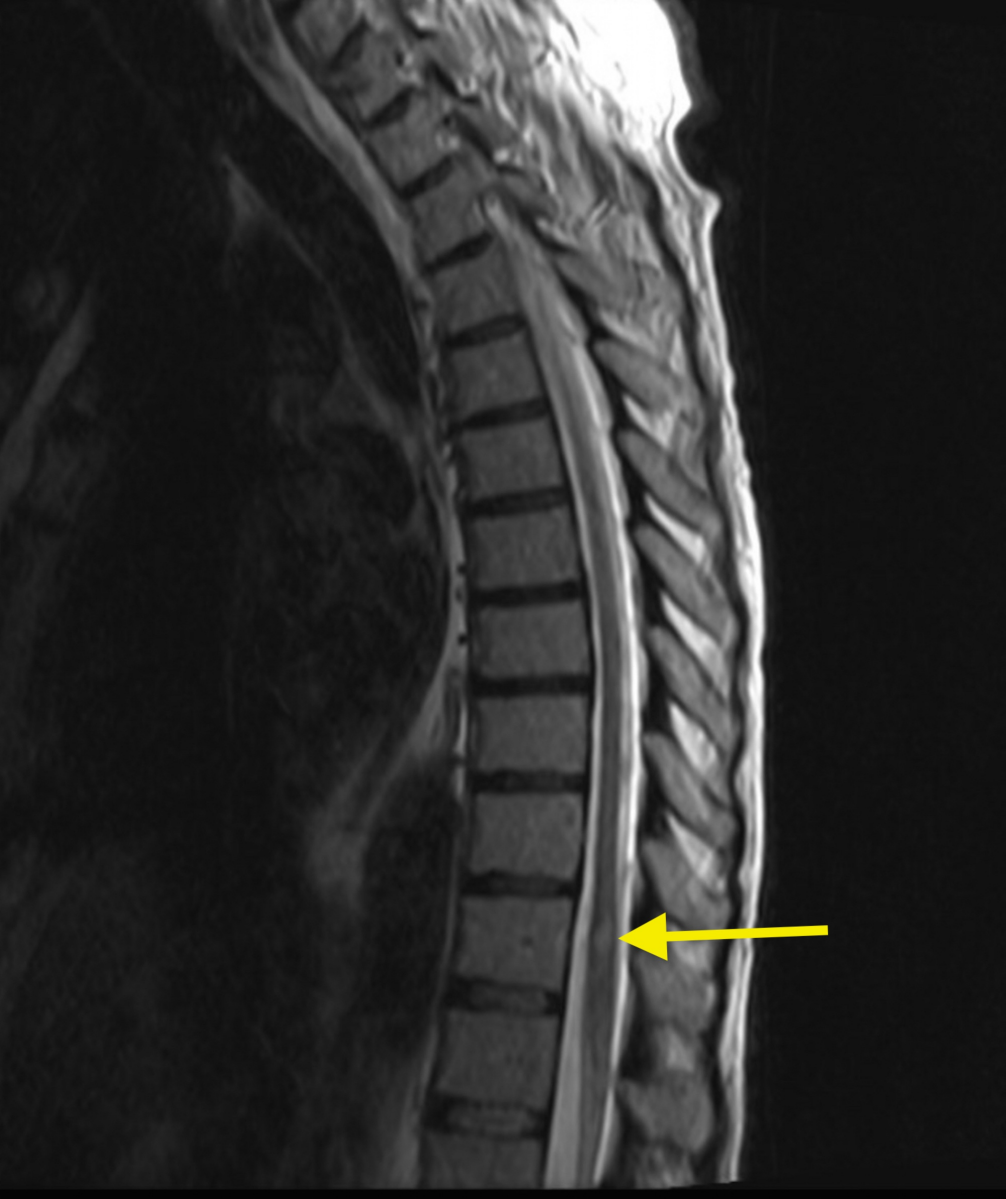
Magnetic resonance imaging (MRI) of the thoracic spine showing hyperintense lesion at T11 level (yellow arrow).
